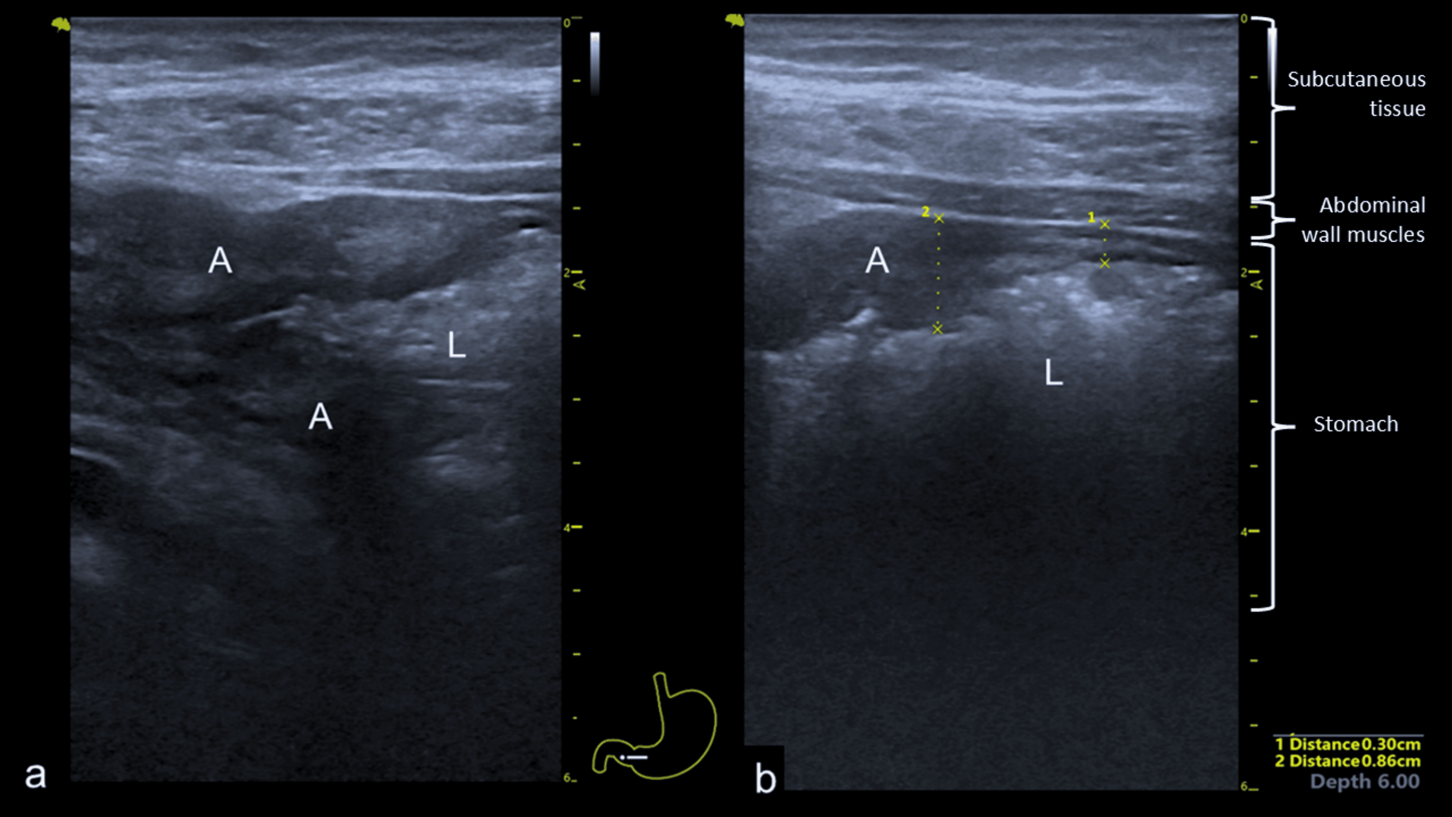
Bedside POCUS showing thickened antrum - a) distal antrum (A) with narrowing of the gastric lumen (L) and b) more proximal antrum without lumen compromise. There is varying wall thickness measuring 8.6 mm at the tumor area and 3.0 mm at the normal part of the stomach. POCUS: point-of-care ultrasound, A: antrum, L: gastric lumen.
